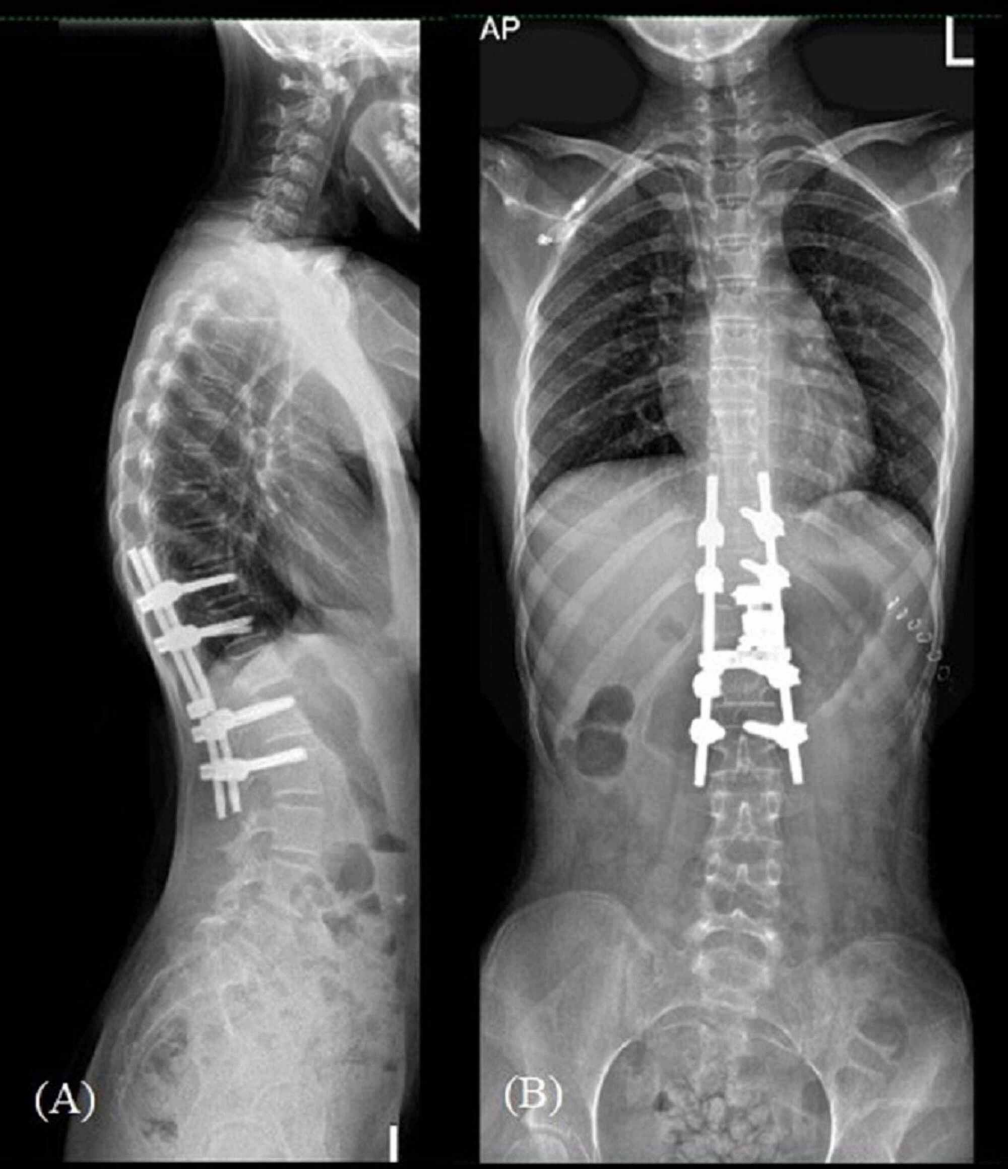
Immediate radiograph post definitive vertebrectomy and spinal fusion.
